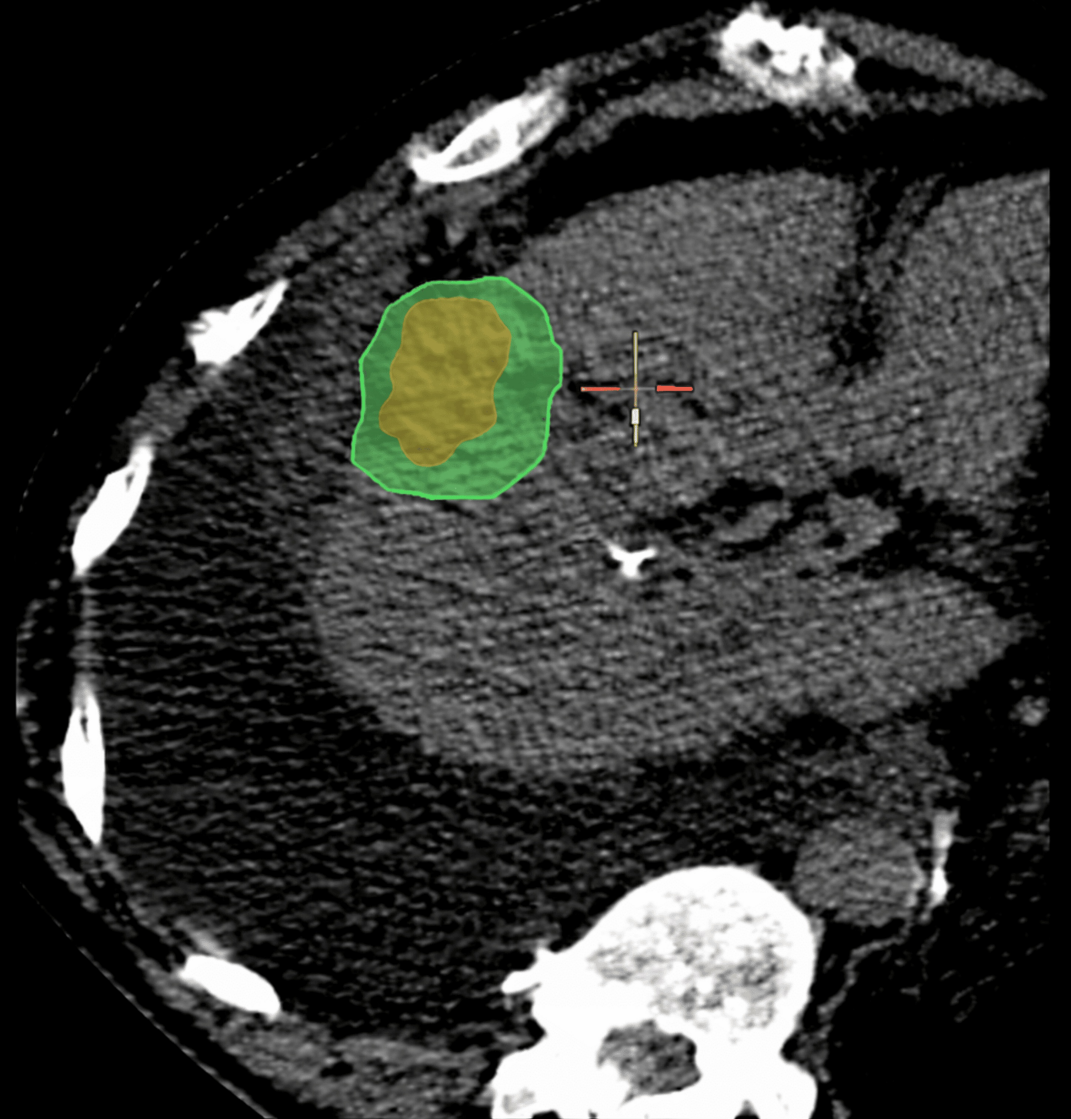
Post-ablation contrast-enhanced computed tomography (CT) demonstrating adequate coverage of the target lesion with appropriate ablation margins, confirmed using image fusion software
